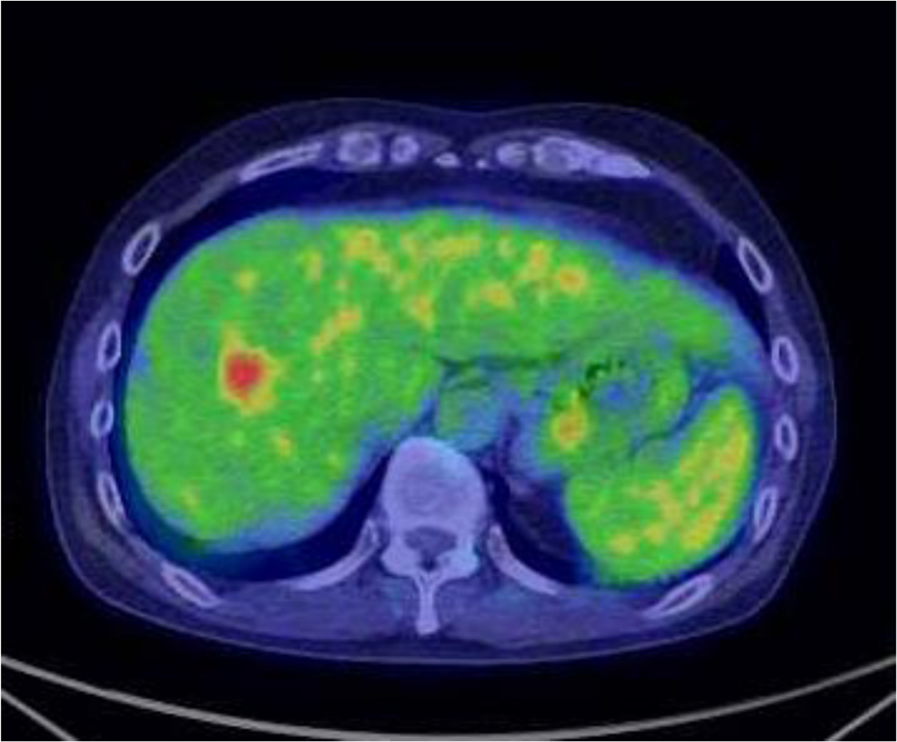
Positron emission tomography-computed tomography after six courses of adjuvant chemotherapy. Imaging showed abnormal accumulation (maximum standardized uptake value of 5.8) in segment 8
